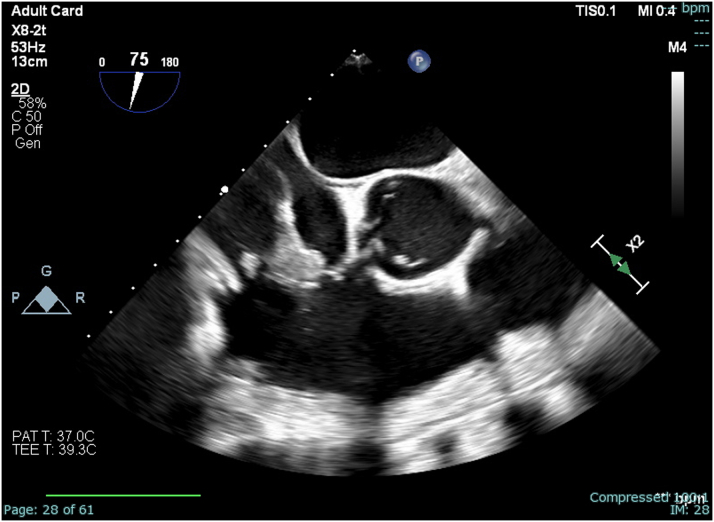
Midesophageal Right Ventricular Inflow-Outflow View During Procedural Transesophageal Echocardiography Thrombectomy catheter is engaged with thrombus midaspiration.
